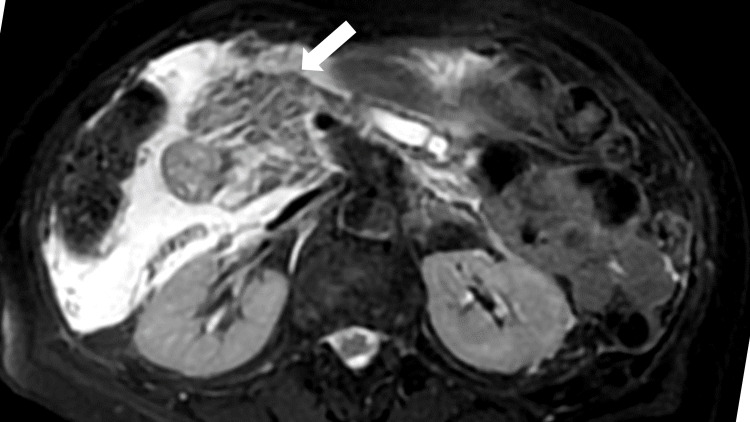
Magnetic resonance image showing an enlarged pancreatic head (white arrow)
